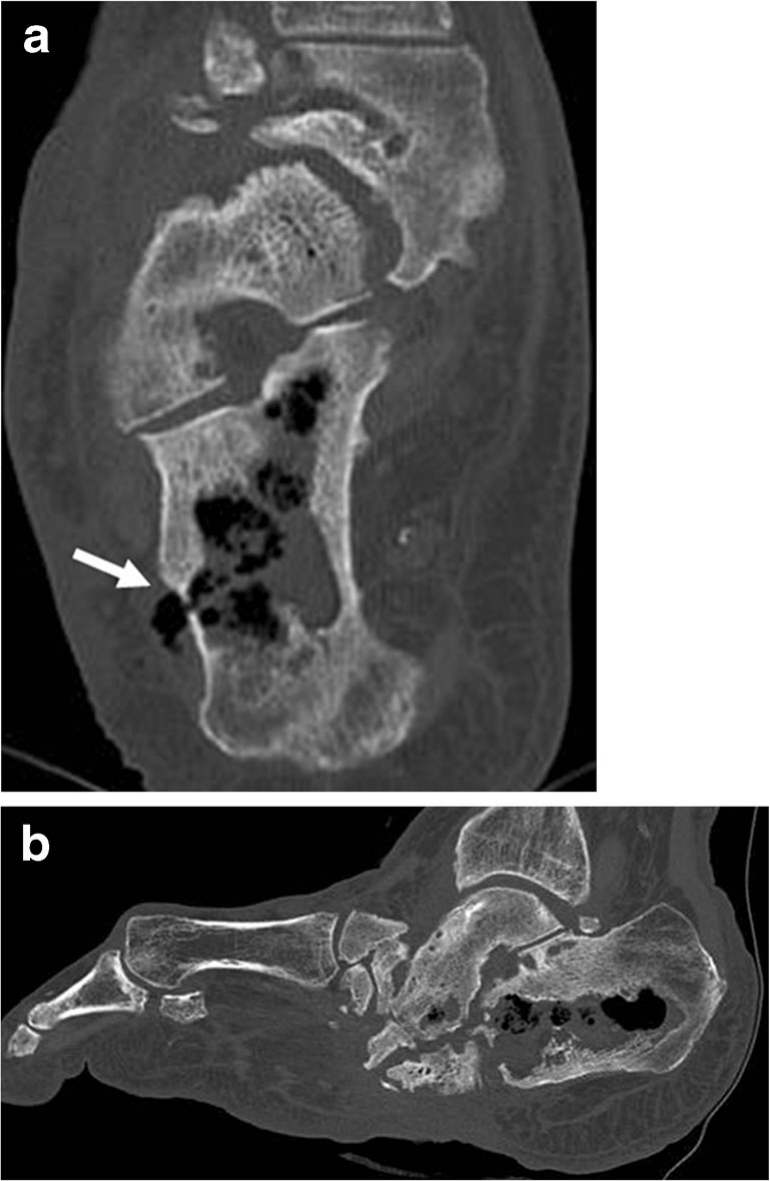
a, b: Unhenanced CT axial (a), sagittal plane. Osteomyelitis of the tarsus in a 72-year-old male with chronic renal failure under dialysis. Extensive bone alterations involving all the tarsal bones with important areas of ostelysis associated with areas of osteosclerosis. The infection extensively involves the calcaneus with intraosseous fluid and gas components. A fistula with subcutaneous soft tissue is visible on the lateral side of the calcaneus (*arrow*)
